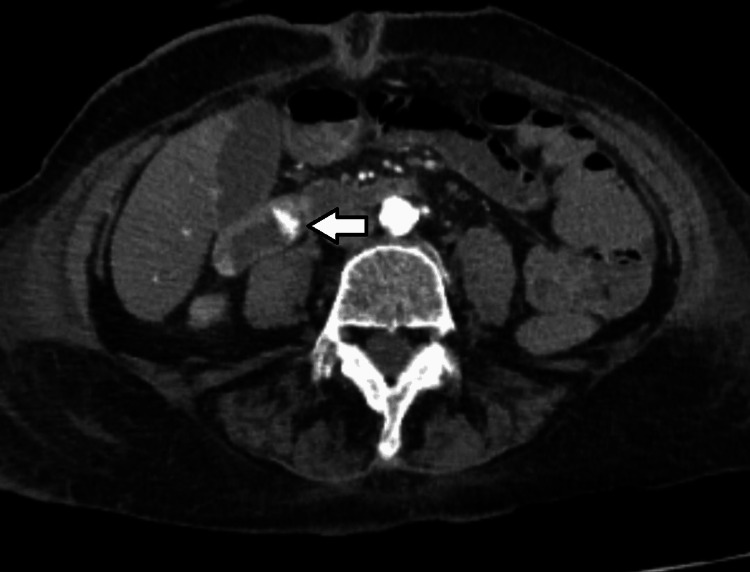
Axial CT of the abdomen Selected axial CT image of the abdomen showing a hyperenhancement focus within the duodenum (arrow) in the arterial phase.
